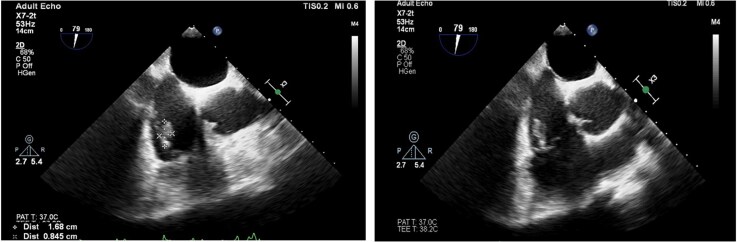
Transoesophageal echocardiography images of the patient. White arrows indicate a mass suspicious for vegetation at the lead tip.
